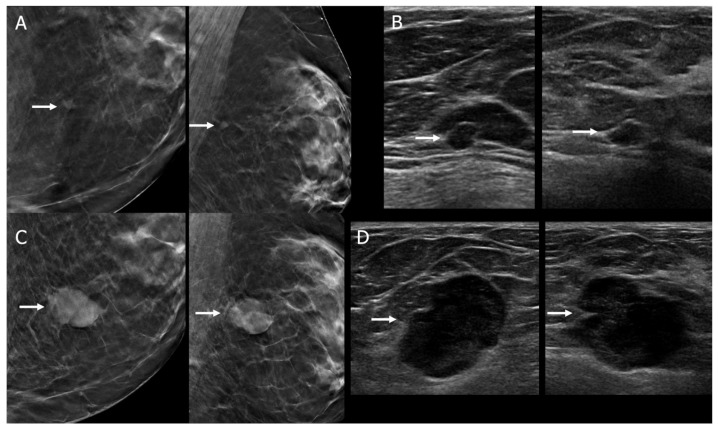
Potential earlier diagnosis of triple-negative invasive ductal carcinoma (TNBC) by AI. This 54-year-old woman was recalled from screening tomosynthesis for a new nodule upper inner left breast. (A) Close-up craniocaudal (CC) and mediolateral oblique (MLO) tomosynthesis images of the left breast show a 6-mm circumscribed mass (arrows) in the upper inner left breast, new compared to prior mammograms (not shown). (B) Targeted radial and antiradial US images show a corresponding circumscribed oval 5-mm anechoic mass (arrows) left breast 11 o’clock position 5 cm from the nipple, with no internal vascularity (Doppler not shown), thought to be a simple cyst, BI-RADS 2. This mass was assessed as suspicious on KDS training (score of 0.57). (C) Close-up CC and MLO tomosynthesis images from screening mammogram left breast, 18 months later, show moderate enlargement of the now lobulated mass (arrows), now measuring 22 mm on (D), radial and antiradial US images. This proved to be a 2.4-cm grade 3 TNBC that showed partial response to neoadjuvant chemotherapy. One sentinel node was negative for metastatic disease.
